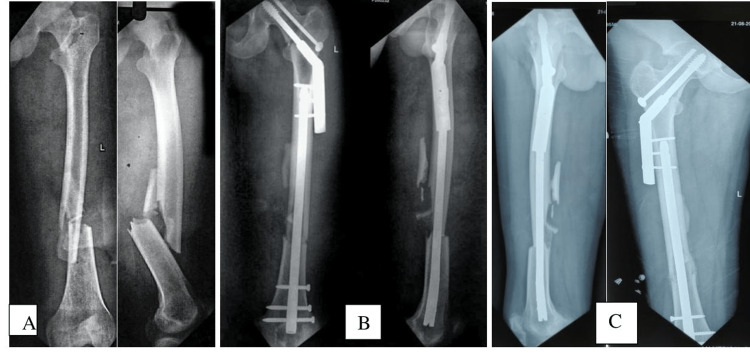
A) preoperative image; B) an immediate postoperative radiograph with good reduction and internal fixation with plate combinations; C) A four-month postoperative radiograph showing a union of the intertrochanteric and femoral shaft fractures
